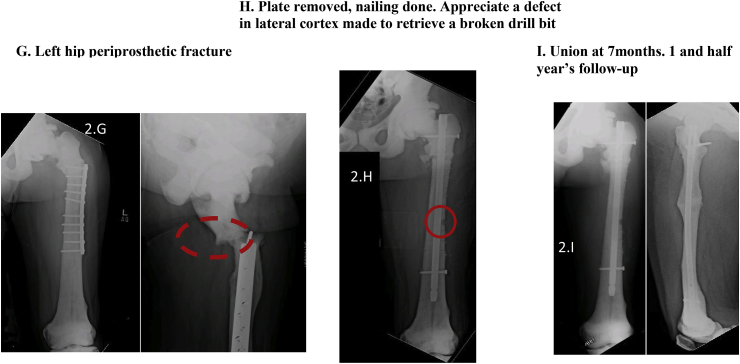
Radiographs of case 2 (next 3 procedures).
